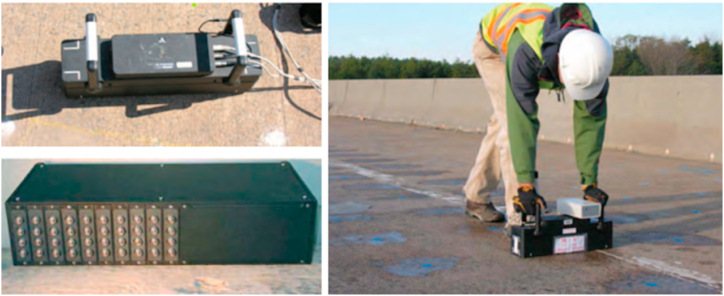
Bridge deck surveying using ultrasonic equipment (Gucunski et al., 2013) [24].
